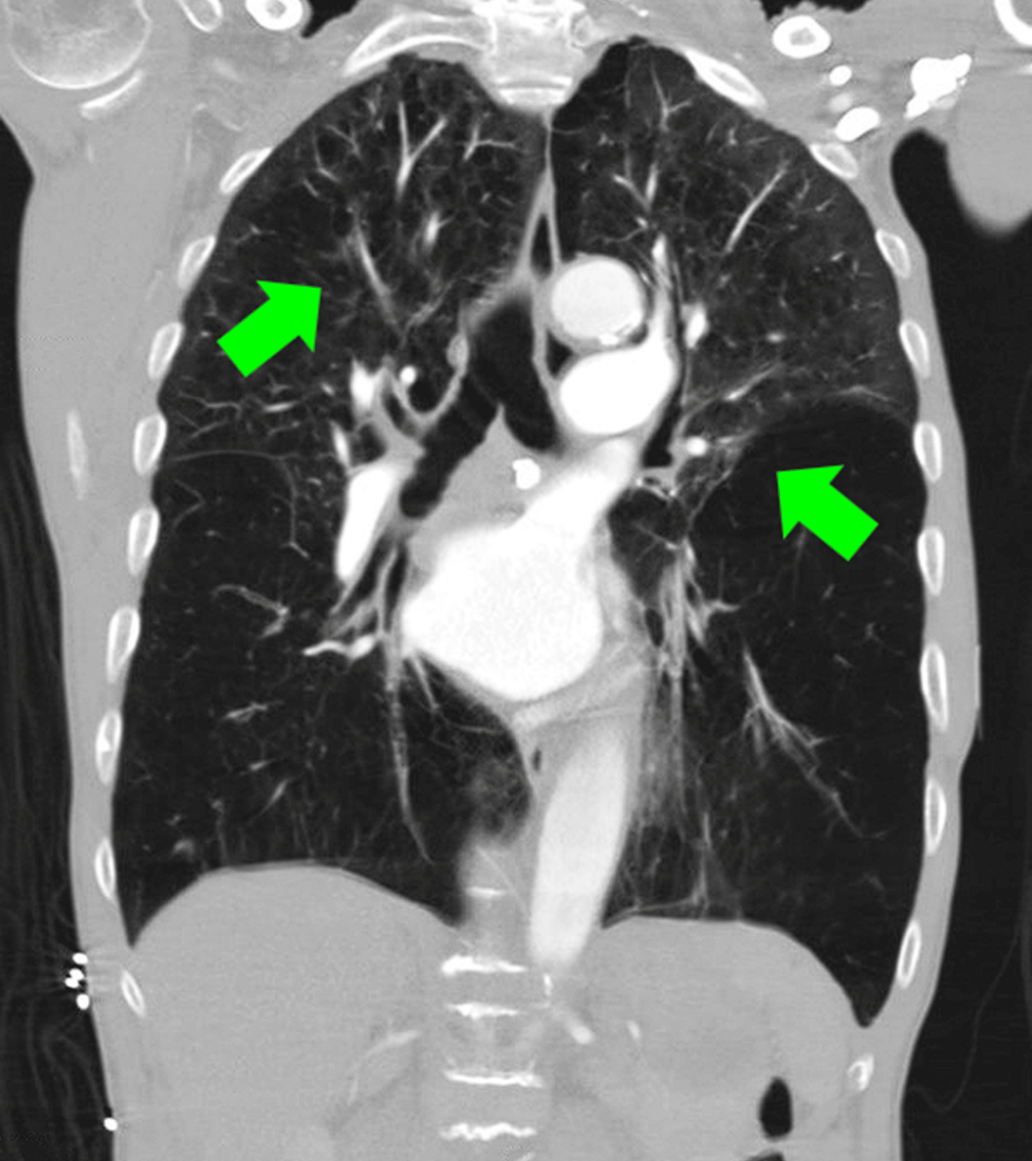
Coronal chest CT angiography (CTA) on admission showing chronic severe emphysema and bronchitis (green arrows).
